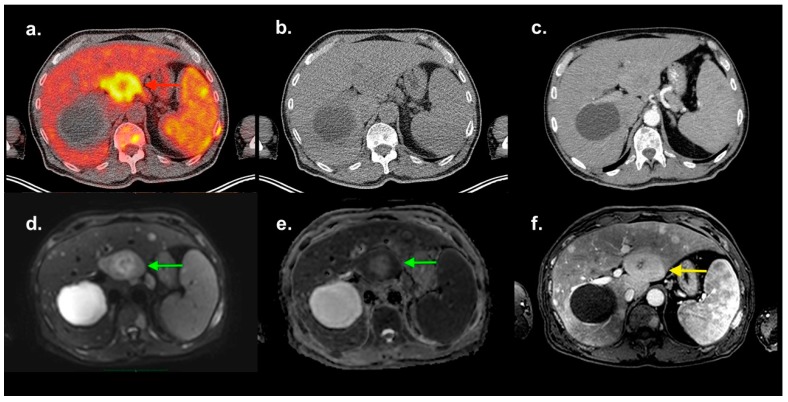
Newly diagnosed patient with multiple myeloma (MM, Typ IgA Kappa, Stage IIIA according to the Durie–Salmon staging system, ISS III), anemia and hepatosplenomegaly underwent an 18F-FDG PET/CT and a routine whole-body MRI investigation, as it is standard for all MM patients. The transaxial fused PET/CT image (a) shows a circumscribed lesion in the IVb segment of the liver (red arrow) with an SUVmax of 10.1, a functional volume of approx. 90 ml and a hypometabolic center. At the same level, a cystic liver lesion of 9 cm in diameter is delineated (without FDG-uptake). The FDG-avid lesion is not distinguishable in the low-dose CT (b). The contrast-enhanced diagnostic CT (c) shows a contrast uptake in the arterial phase nearly similar to the liver parenchyma, and a hypodense core demarks itself as in the PET/CT-image. Diffusion-weighted (DW d,e) MRI demonstrates an inhomogeneous abnormal diffusion restriction in the same area (green arrows), which can be clearly distinguished, showing an intermediate T1-signal intensity and a hyperintense core (yellow arrow) in the contrast-enhanced MRI (f). A biopsy of the lesion under sonographic control indicated plasma cell neoplasia. A diagnostic ultrasound of the liver was not performed before (no pre-described MM hepatic disease). The presence of extramedullary lesions at any time during the course of MM indicates a poor prognosis [1], and these patients may need to follow additional treatment strategies [2,3].
